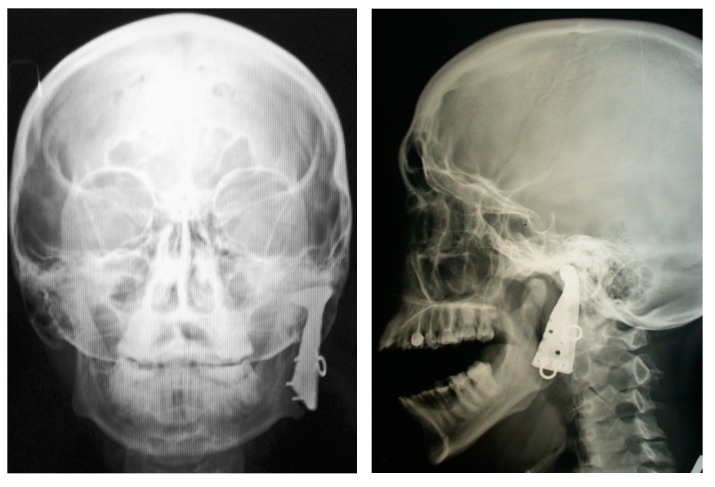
X-ray images of the repaired left mandible for deforming temporomandibular osteoarthrosis (a year after surgery) [102].
